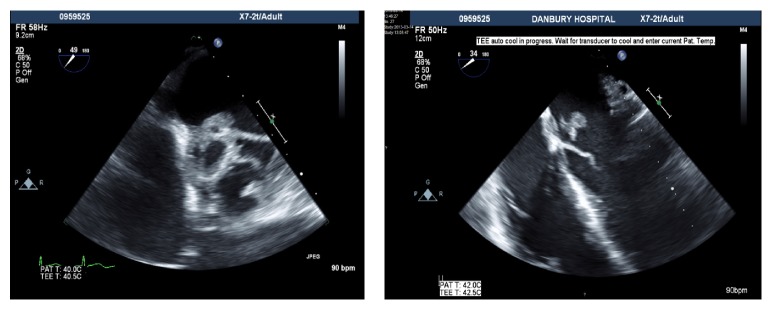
Transesophageal echocardiogram. Mass seen on anterior leaflet measuring 1.2 × 1.8 cm with perforation of the leaflet. Bicuspid aortic valve with severe aortic regurgitation and abscess cavity and fistula extending from right coronary cusp to left atrium.
